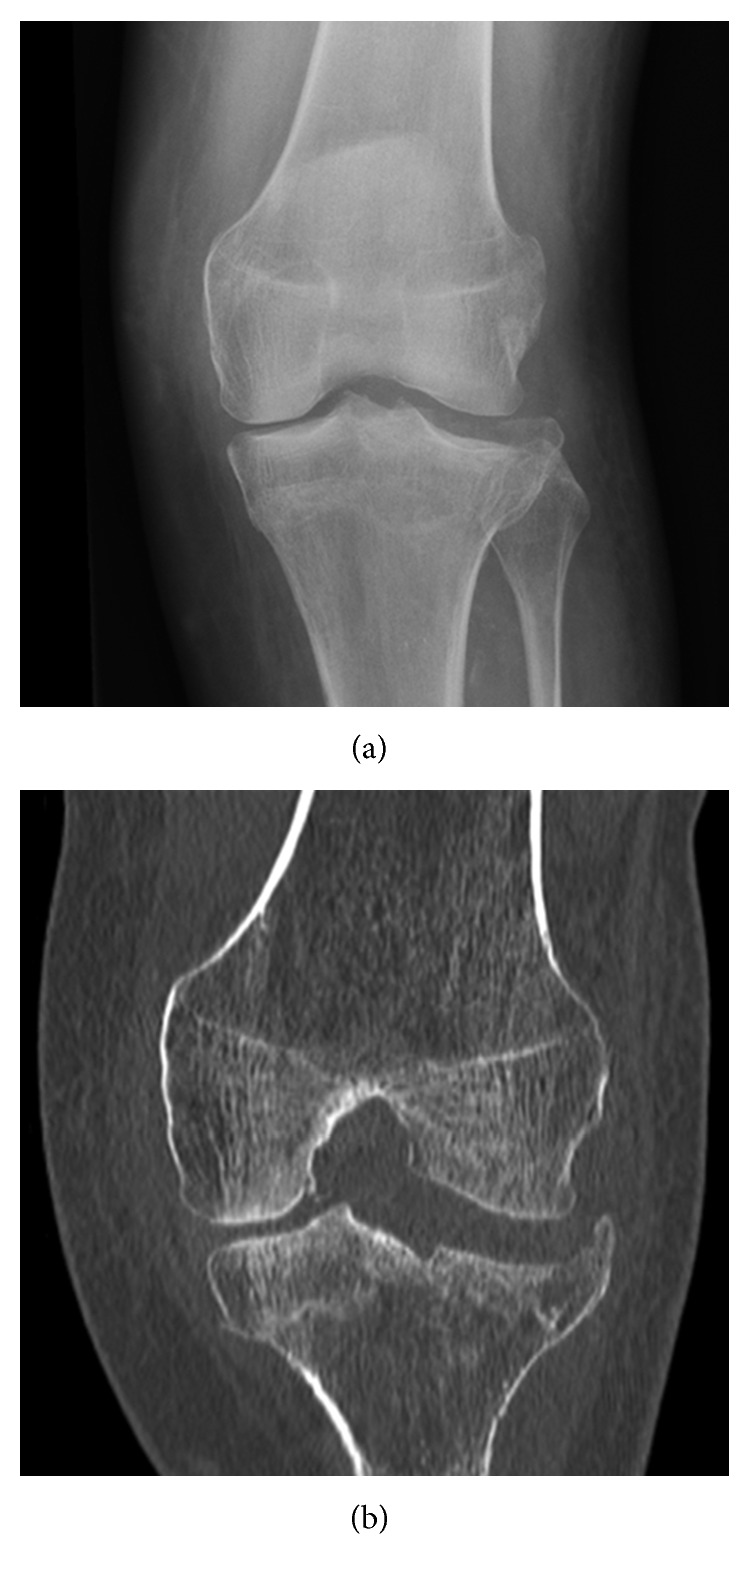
Patient 2 initial presentation. (a) Anterior-posterior radiograph and (b) coronal CT scan.
